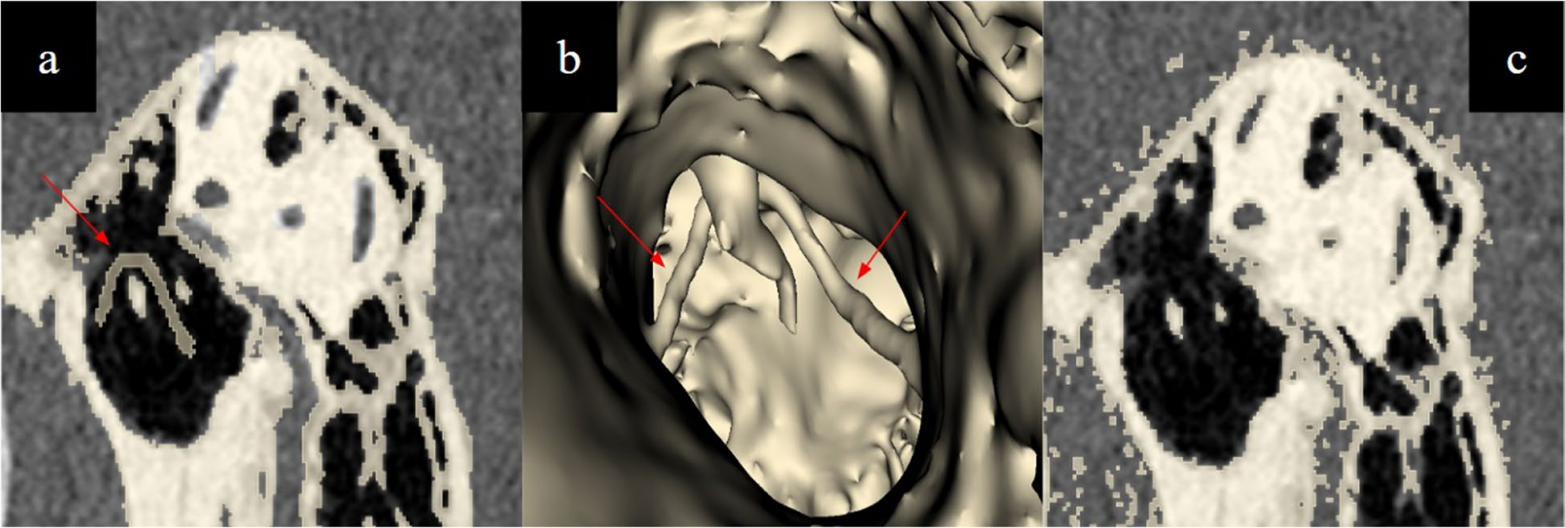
a CAD modelling and (b) 3D rendering of the expected course of the tympanic segment of the chorda tympani nerve (arrow) which courses medial to the handle of the malleus. c This is not normally visualised on CT due to its thin size and low density. The nerve is identified and preserved during ENT procedures involving the middle ear
